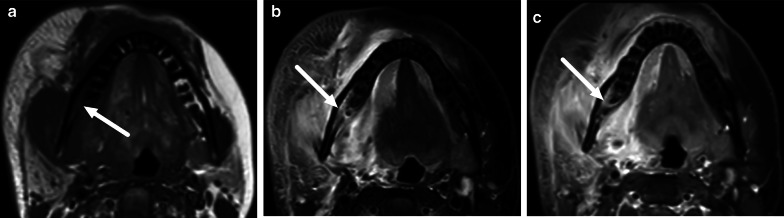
Bone marrow edema as evidenced by low T1-signal (a) and high signal on fat-suppressed T2-weighted (b) and post-contrast T1-weighted (c) axial images (arrows) reliably suggest an odontogenic origin of infection. Image modified from ref. [11]
